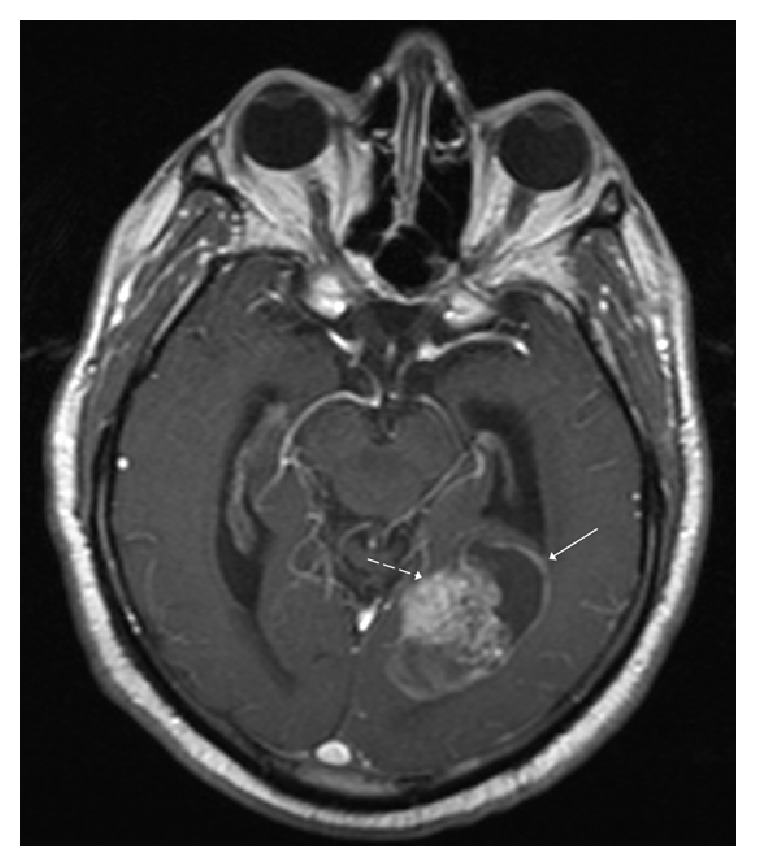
Postcontrast axial T1 weighted MR image demonstrates avid heterogeneous enhancement in the solid component (dashed white arrow) with rim enhancement of the cystic component.
